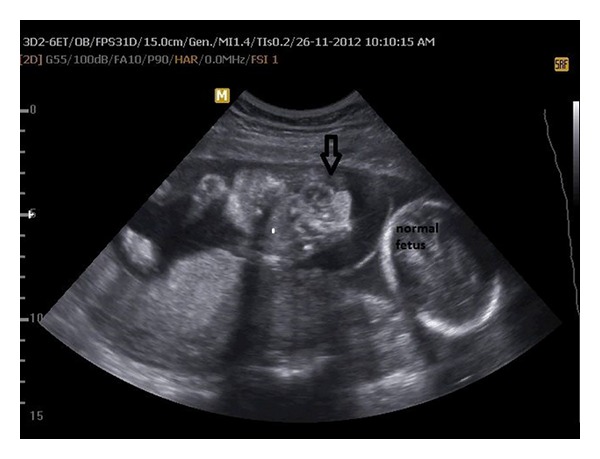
Ultrasonographic view of twins at 23 weeks of gestational age; arrow indicated heart protruding out of the sternal defect.
